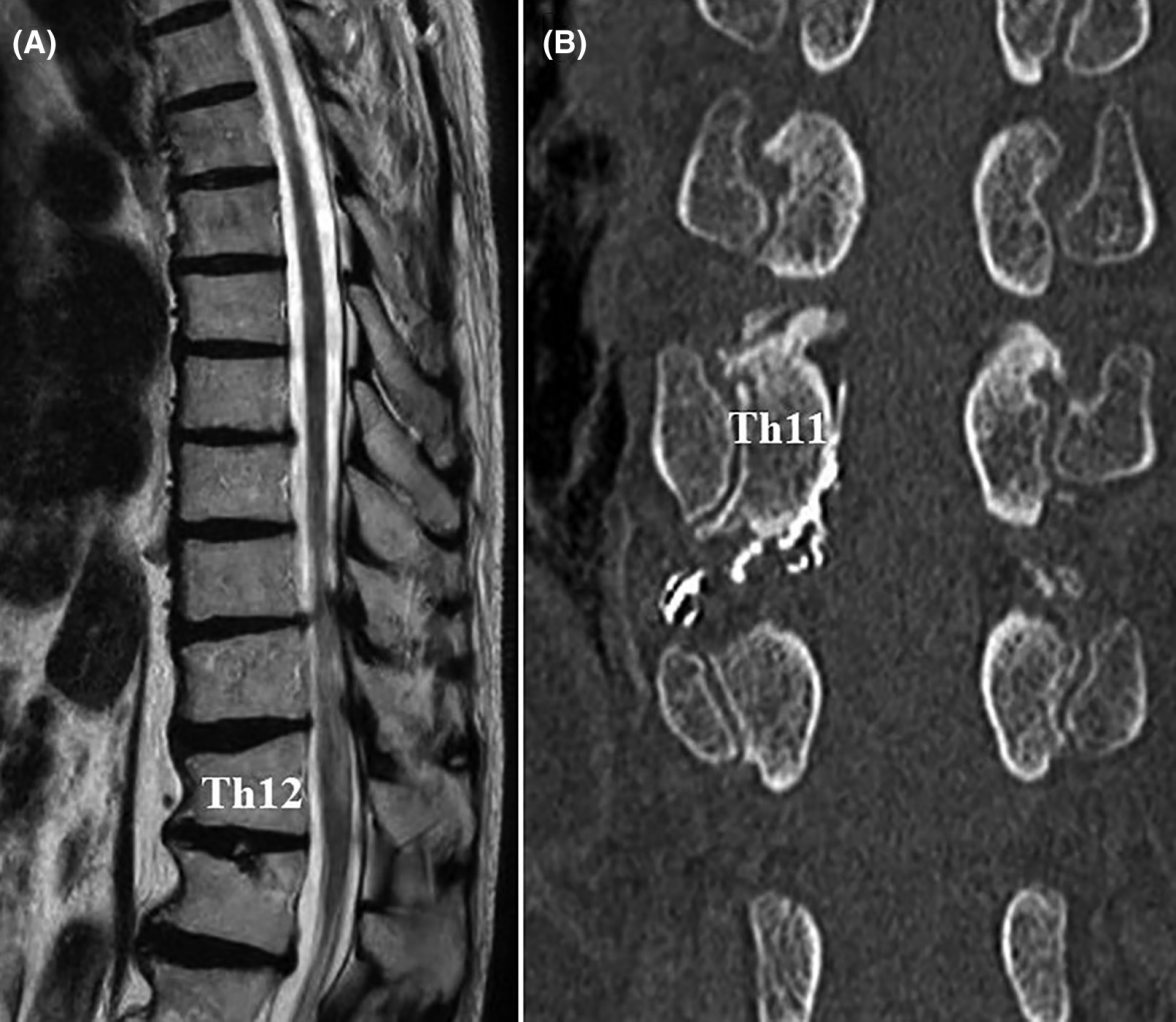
(A) Post‐treatment sagittal T2‐weighted MRI of the thoracolumbar spine showing the disappearance of edema and flow voids. (B) Coronal computed tomography revealing n‐butyl‐2‐cyanoacrylate at Th11/12 vertebral foramen.
